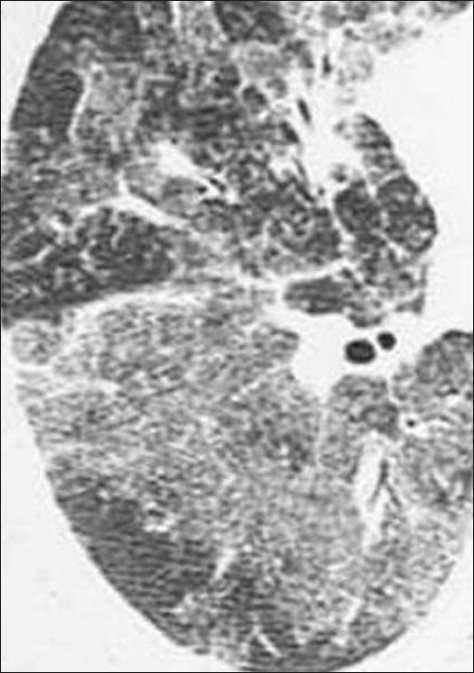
Section of an HRCT showing amiodarone lung. N ote the high density lung, septal thickening and features of interstitial fibrosis
